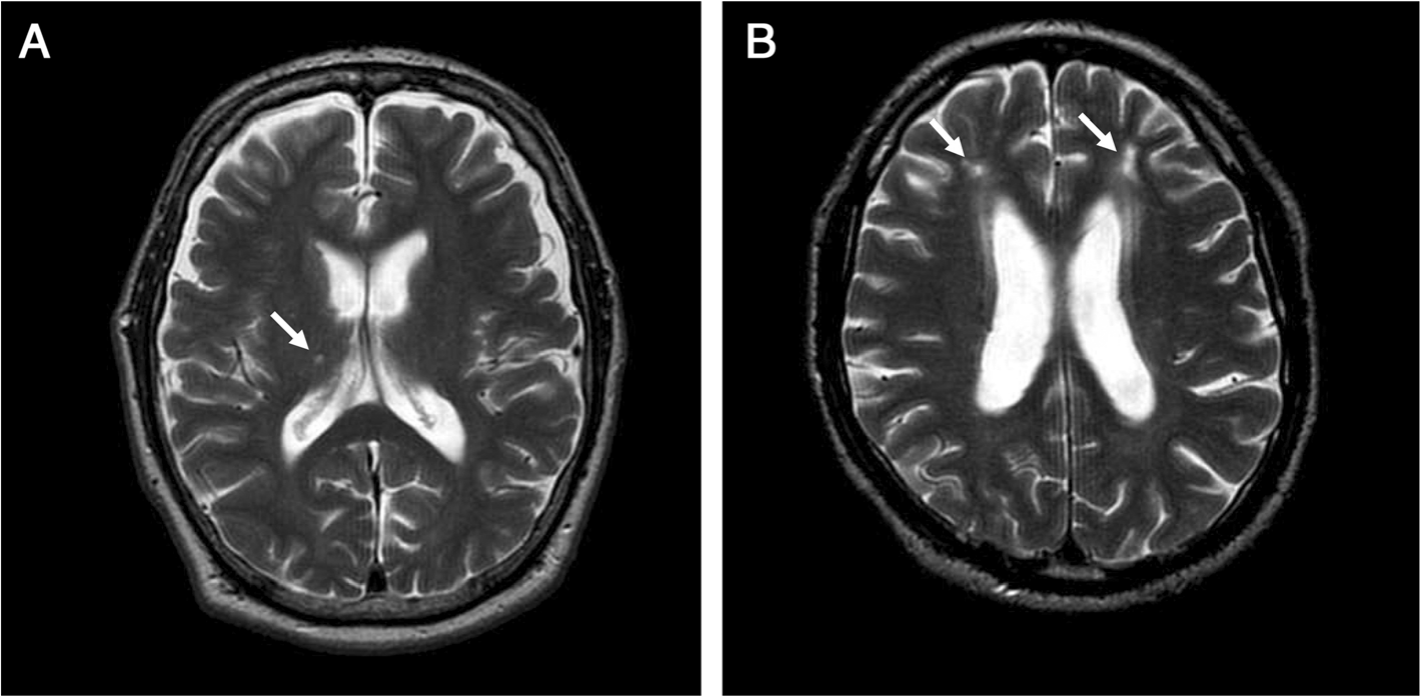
Brain MRI. Representative brain MRI findings (T2-WI) of non-diabetic (A) and diabetic (B) nephropathy patients are shown. Arrows indicate cerebral infarctions.
